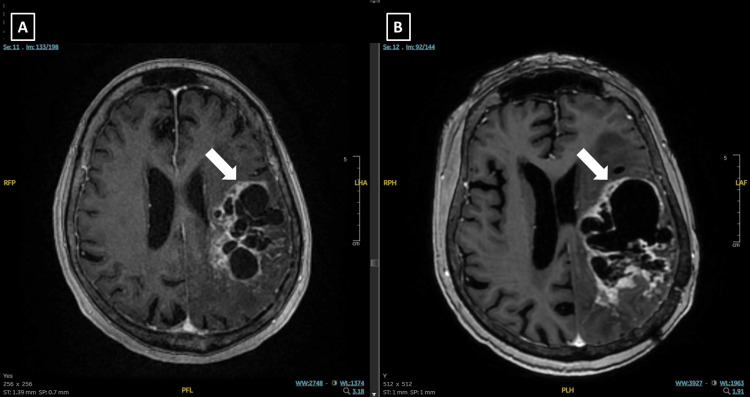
Diagnostic and 3-month follow-up MRI images of Case 1. (A) Axial T1-weighted post-contrast (T1C+) MRI at diagnosis showing a solid contrast-enhancing mass with cystic components in the left temporal lobe, measuring 6.1 × 4.4 cm. (B) Axial MRI at the 3-month follow-up after LATTICE radiotherapy. The lesion shows an increase in cystic components, with persistent T2 hyperintensity and more prominent contrast enhancement compared to the 1-month post-treatment scan.
